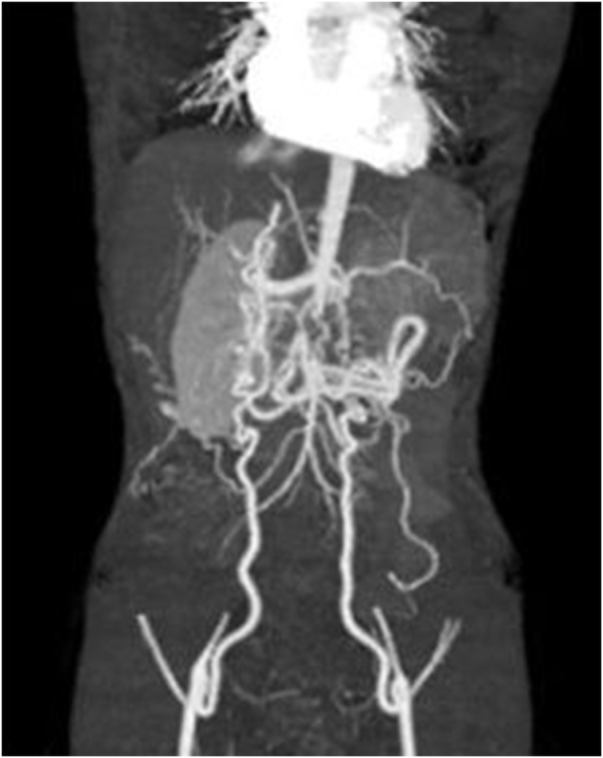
Initial computed tomography angiography of the patient. Long-segment coarctation of the abdominal aorta is demonstrated extending from below the renal arteries to just above the aortic bifurcation. Marked stenosis of the left renal artery is evident, with the left kidney appearing atrophic and poorly perfused. Extensive collateral circulation is visualized, suggesting a chronic and critical compromise of distal aortic flow, consistent with severe mid-aortic syndrome.
